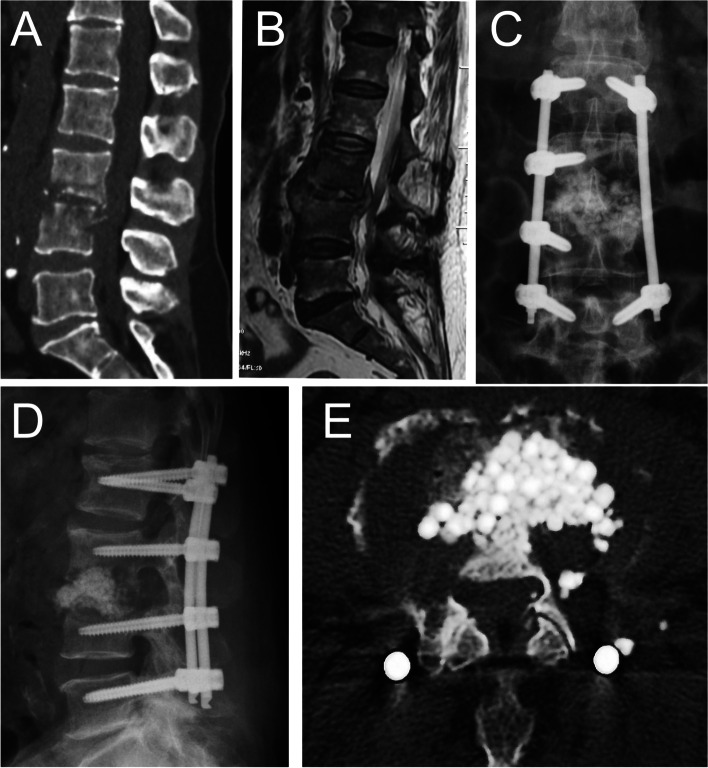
A 65-year-old female suffered from low back pain with lumbar spondylodiscitis. A Preoperative sagittal CT showed L3/4 disc involvement and bone defects. B MRI showed abscess formation which involves the spinal canal. C,D Postoperative anteroposterior and lateral radiographs after short segmental fixation showed good spinal alignment. E Postoperative axial CT showed the intervertebral space is filled with antibiotic-loaded calcium sulfate beads
